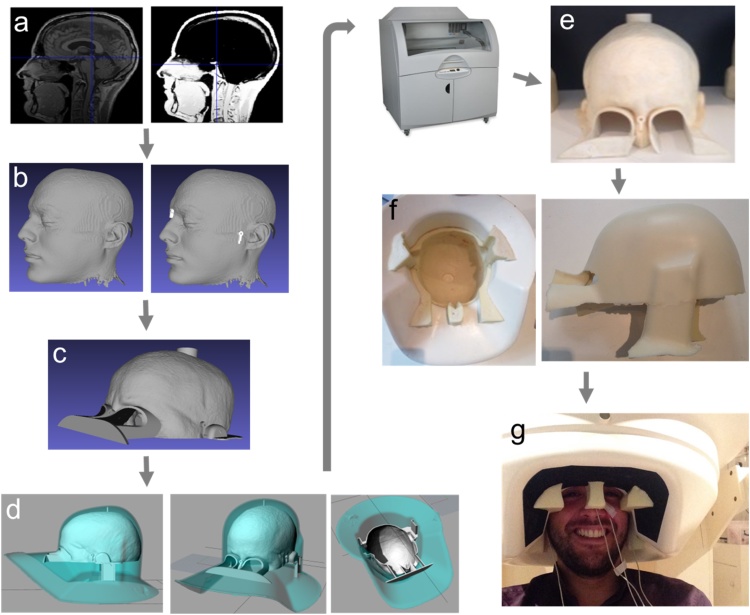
Overview of head-cast construction steps. a) Head surface is extracted from an anatomical MRI image using the standard SPM12 segmentation procedure. b) Head surface extraction is converted to a surface file and fiducial coils are added. The coil locations are defined in MRI coordinates. c) A positioning cylinder at the top of the head is added to the virtual model to define the position of the head inside the head-cast. Eye extensions are added to enable vision during use. d) Using and adjusting the positioning cylinder, eye extensions and ear extensions, the virtual head model is positioned appropriately inside a virtual copy of the MEG dewar. e) The positive head model is 3D printed. f) The 3D print is placed inside the manufacturer-provided dewar copy (as in d) and foam resin is poured in to fill the gap between the printed positive head model and the dewar. The fiducial coil protrusions on the 3D printed head result in coil-shaped and coil-sized indentations in the head-cast (the nasion coil protrusion is visible between the eyes in e). g) The subject can now wear the flexible foam head-cast and enter into the (real) MEG dewar for scanning.
